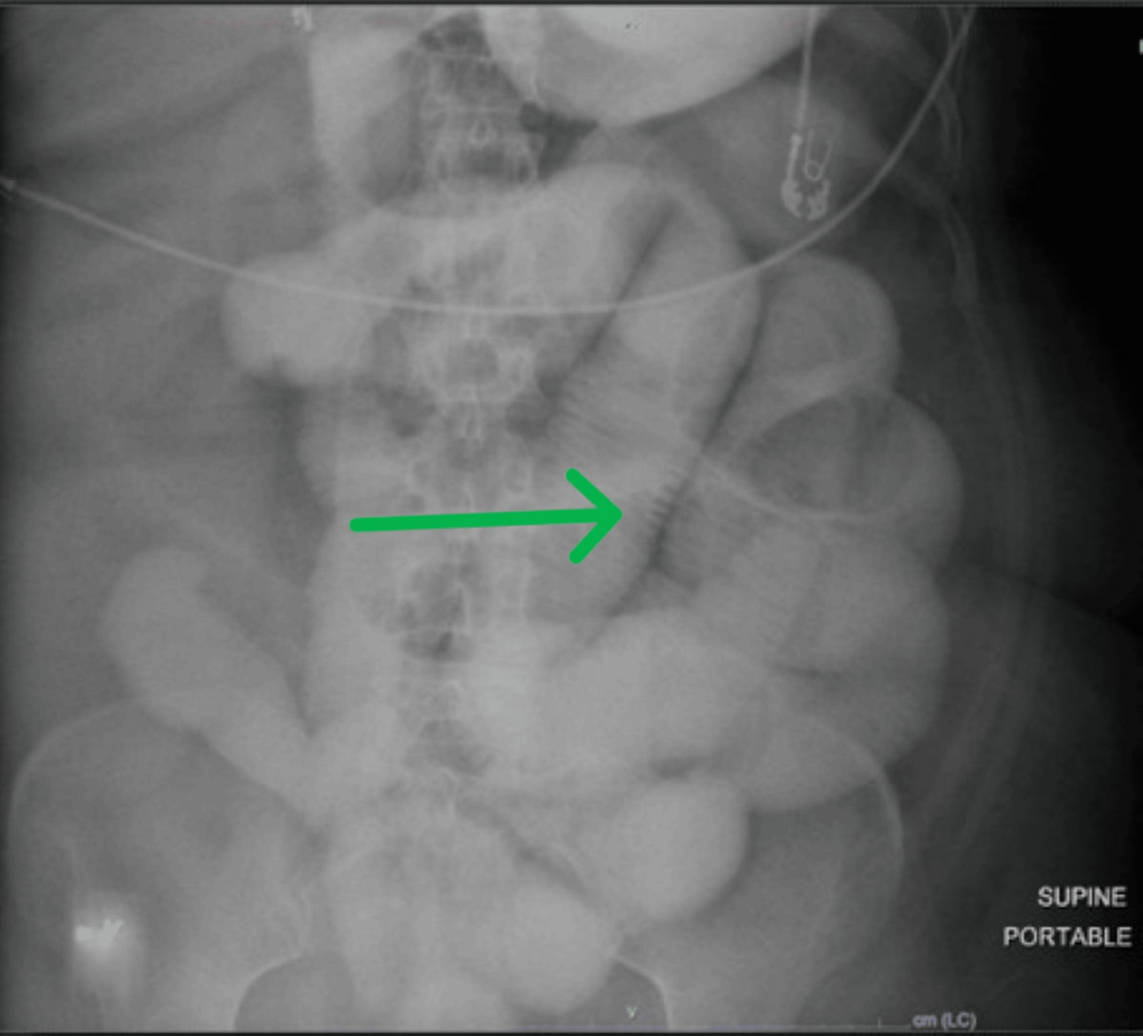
X-ray of the small bowel follow-through 24 hours after oral gastrografin study demonstrating persistent contrast in dilated small bowels, with the arrow pointing at valvulae conniventes, classic for small bowel distension, indicating high-grade small bowel obstruction.
